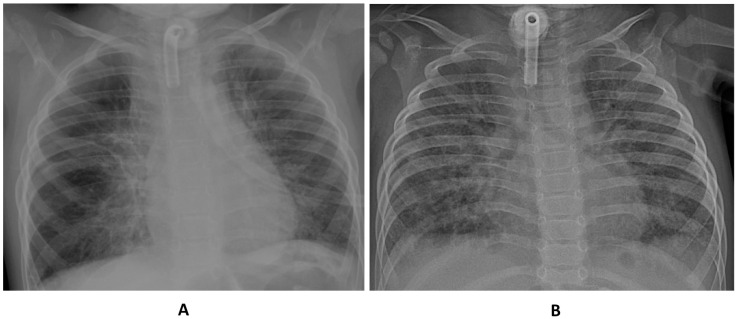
Chest X-ray pulmonary aspect before infusion 1 (A) and infusion 5 (B) of mesenchymal stromal cells.
